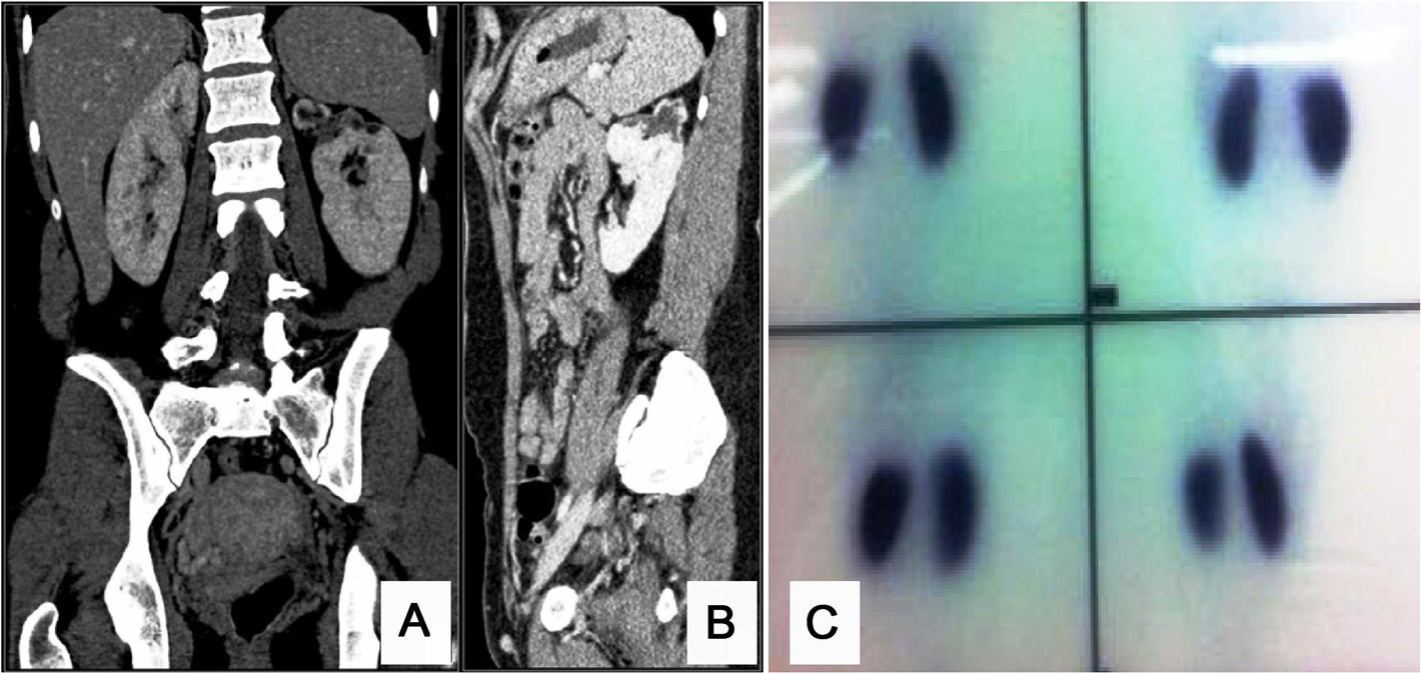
Computed tomography and V/Q scan after treatment. Coronal (A) and sagittal contrast-enhanced computed tomography (CT) images (B) reveal the cystic degeneration of the upper pole of the kidney drained by the chronically obstructed ectopic left ureter, ^99m^Technetium- dimercapto-succinic-acid (^99m^Tc-DMSA) renal scintigraphy (C) reveals foto-deficient area, located on the upper pole of the left kidney.
